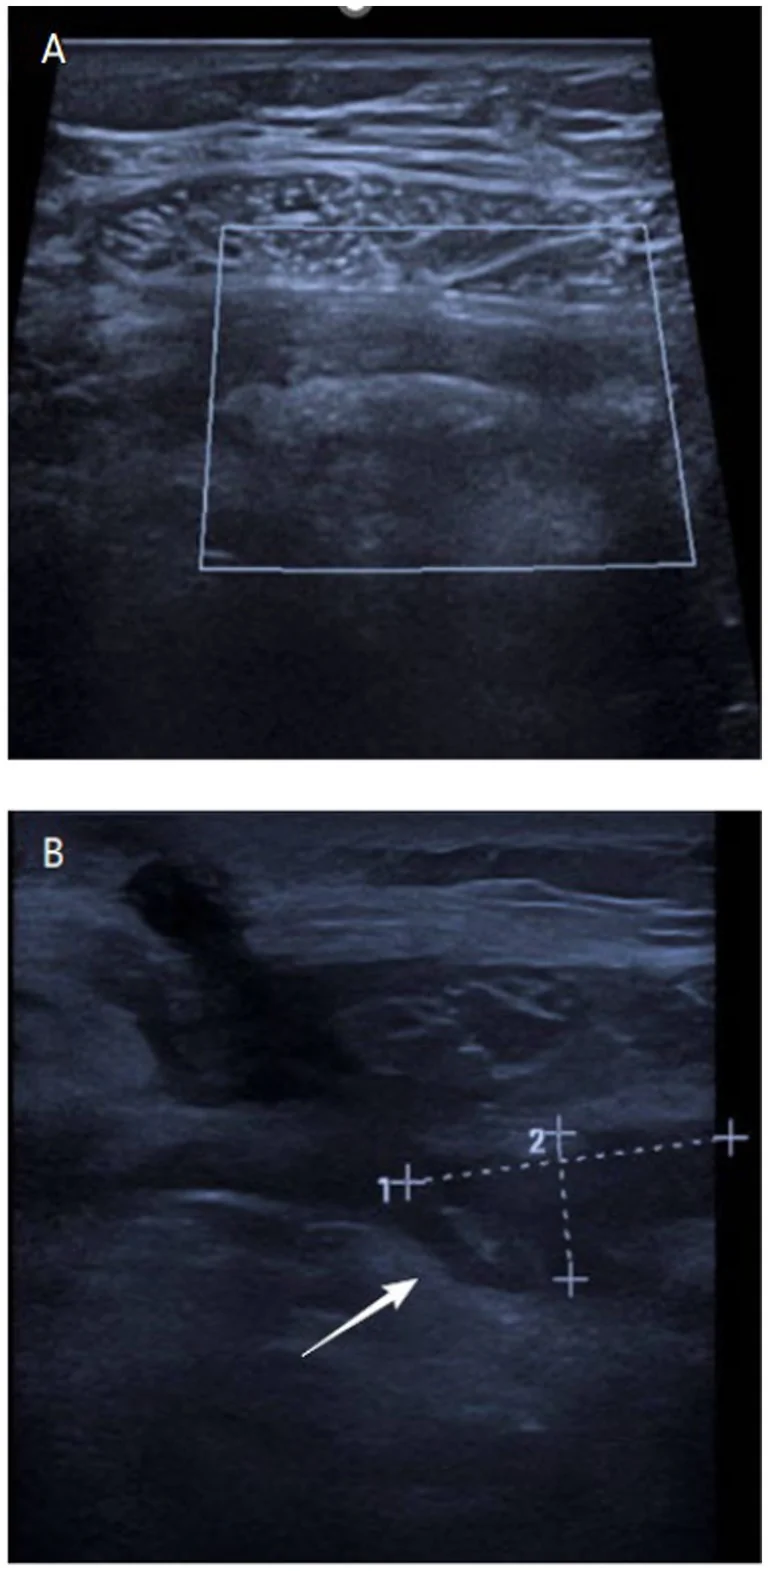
Ultrasound findings during recurrent abdominal collection. (A) On HD30, ultrasonography showed a 27 mm × 7 mm anechoic area with poor transparency and punctate echoes, consistent with localized fluid collection (white box). (B) On HD58, ultrasonography showed a 24 mm × 10 mm anechoic area with poor transparency and punctate echoes communicating with the abdominal wall sinus tract; flow sensation was observed with probe compression (white arrow).
